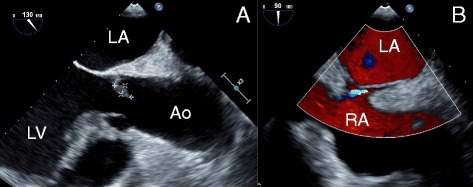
Transesophageal echocardiography findings. Vegetative lesions are visualized at the right coronary cusp (3 × 12 mm) and the noncoronary cusp (6 × 12 mm) of the aortic valve with the presence of newly developed severe aortic regurgitation (a). An interatrial shunt, suggesting the presence of patent foramen ovale, as demonstrated on color Doppler ultrasonography (b). *LA* left atrium, *LV* left ventricle, *Ao* ascending aorta
